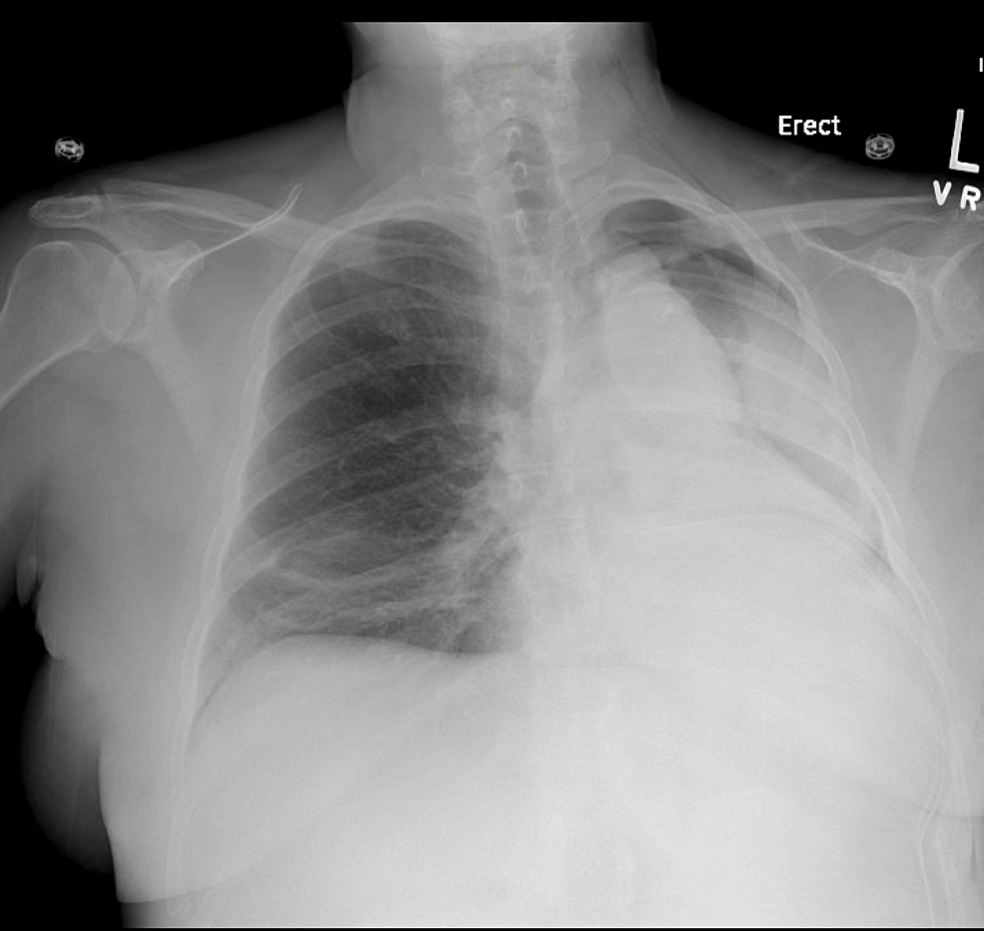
The chest X-ray reveals a large left-sided pneumothorax and total left lung opacification, which is associated with a heart and mediastinal shift to the left.
